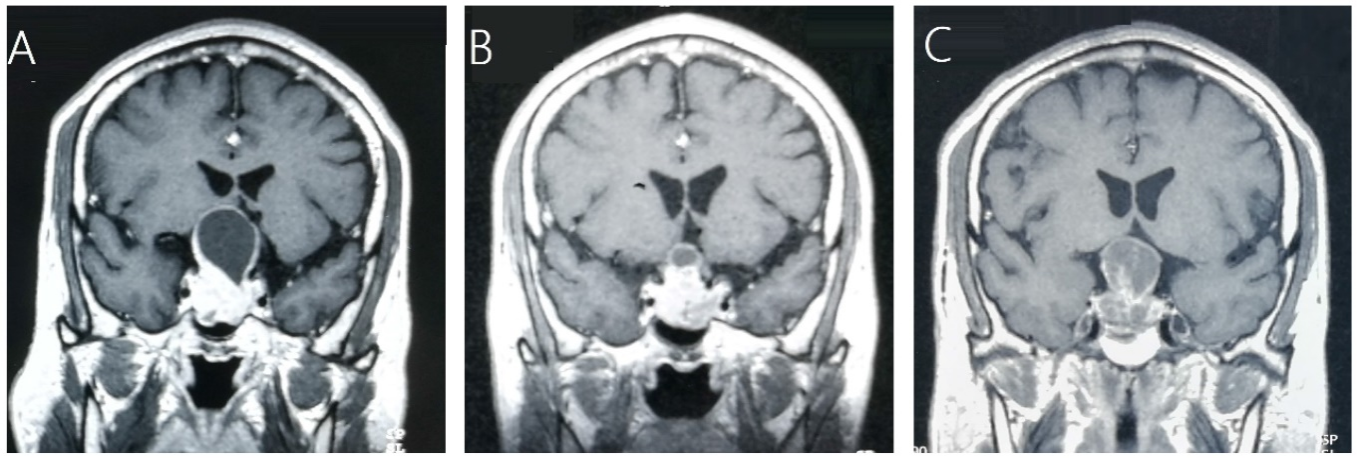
A 45-year-old male patient with residual NFPA received GKRS (10.5Gy/30%) at 4.9 months after surgery and tumor progression due to progressive cystic enlargement was detected at 32.5 months after GKRS. A, MRI showed pituitary giant adenoma in sellar area. B, MRI showed residual tumor at 4.9 months after surgery. C, MRI showed tumor enlargement due to progressive cystic enlargement at 32.5 months after GKRS.
